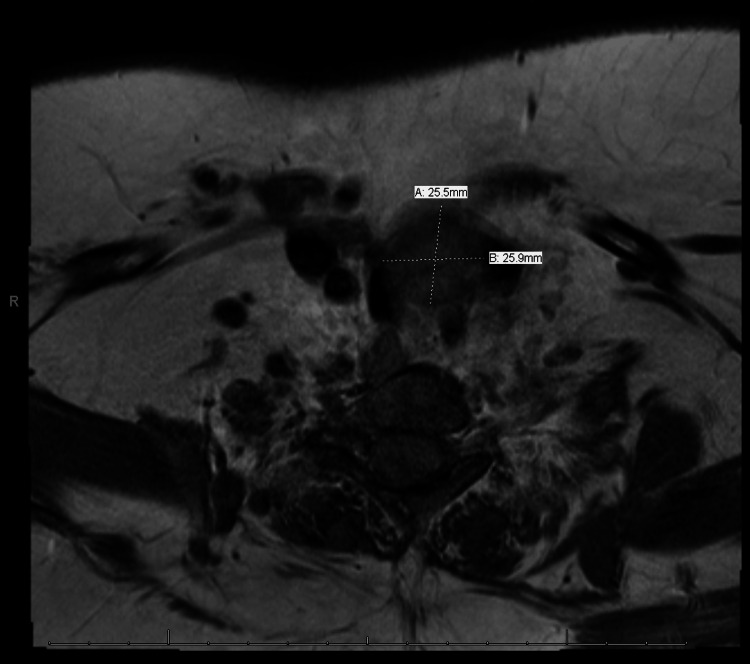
Magnetic Resonance Image of neck with 25.5mm x 25.9mm thyroid nodule.
